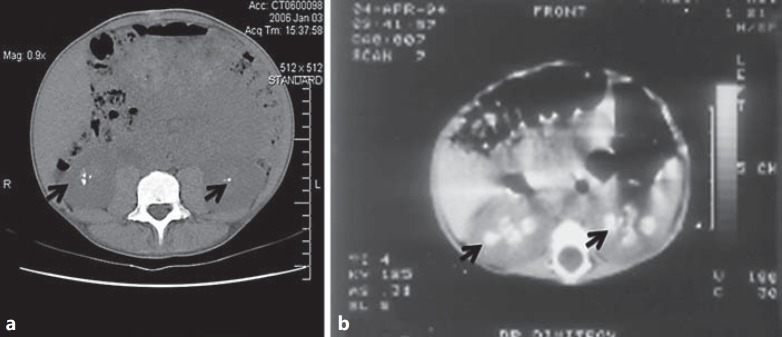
Representative CT images detailing nephrocalcinosis. Shown are abdominal CT images from patient 4, age 24 years (a) and patient 5, age 9 months (b). Note the calcium densities in the kidneys (arrows).
